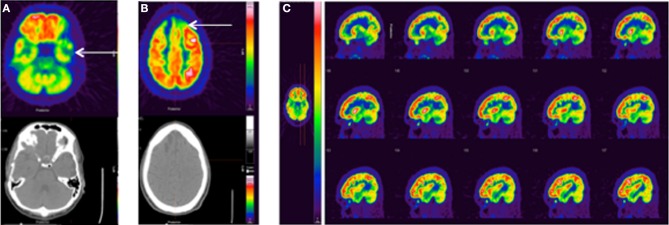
Standard FDG-PET images from three clinical TBI cases. (A) 25 y.o. male, single non-blast moderate TBI, imaged 5 months post-injury. No recorded medications or sleep difficulties, pain/headache, or vision problems. FDG-PET shows left temporal hypometabolism associated with mild volume loss/encephalomalacia on the CT. (B) 28 y.o. male, single non-blast severe TBI, imaged 12.5 months post-injury. No recorded medications or sleep difficulties, pain/headache, or vision problems. FDG-PET shows a more severe injury with prominent hypometabolism frontally (arrow) associated with encephalomalacia. (C) 35 y.o. male, history of repeat exposure to blast-related mTBI, imaged 43 months post-injury. Pain medication (ultram), mild body pain, and moderate sleep problems, no findings CT. FDG-PET shows prominent frontal hypermetabolism, which may be medication related. The color bar displayed in (C) applies to all images with red representing greater FDG uptake.
